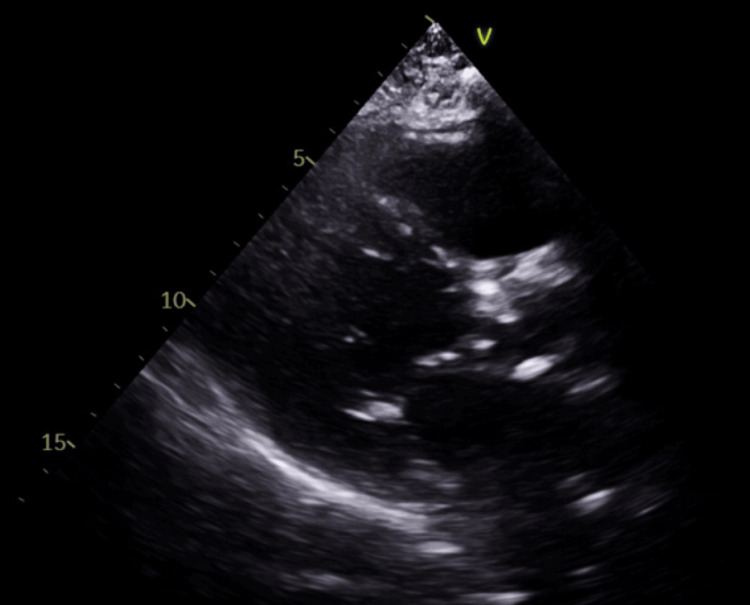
Pre-TAVR Stenosed Aortic Valve Ultrasound parasternal long-axis view: severely calcified aortic stenosis TAVR: transcatheter aortic valve replacement
